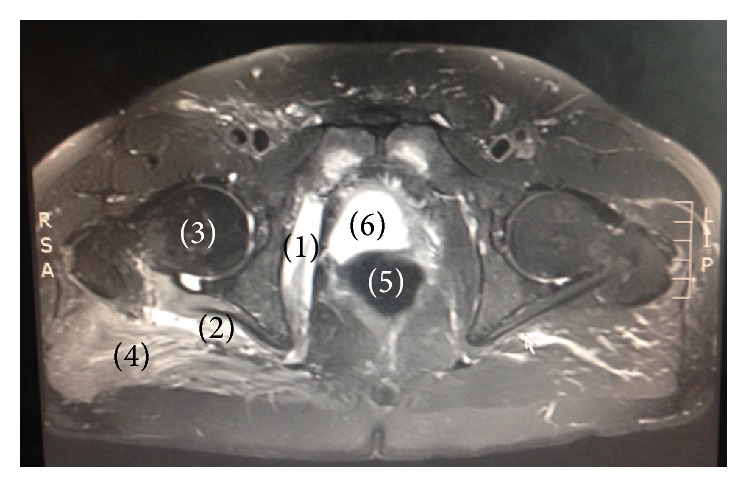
Signal abnormality of the right short external rotators on axial section of T2-weighted MRI. (1) Obturator internus. (2) Quadratus femoris. (3) Head of femur. (4) Gluteus maximus. (5) Rectum. (6) Bladder.
